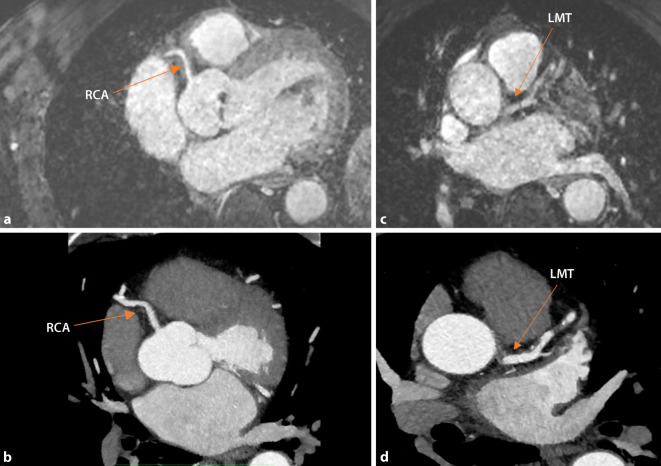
Coronary imaging using coronary CT angiography and coronary magnetic resonance angiography (CMRA). Whole-heart sequence for visualization of the coronary arterial tree. a, b Self-navigated 3D CMRA. Whole-heart sequence (CMRA, a) with corresponding coronary computed tomographic angiography (cCTA, b) acquired from a patient revealing the proximal right coronary artery (RCA). c, d Self-navigated 3D CMRA. Whole-heart sequence (CMRA, c) with corresponding cCTA (d) acquired from a patient revealing the left main trunk (LMT)
